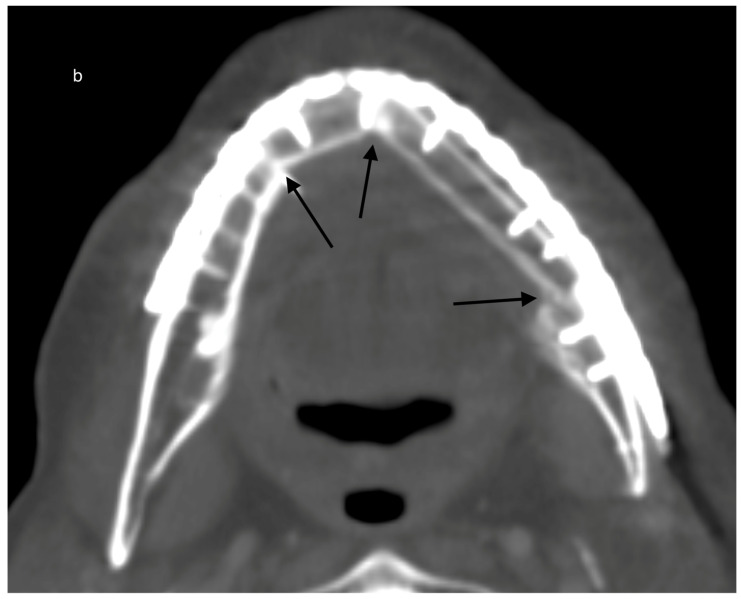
Example for ossification in CT scans over time in a case with a primary reconstructed mandible after malignant tumor resection and post-reconstructive adjuvant radiotherapy (postORT): Example for a reconstructed mandible with a 2-segmented fibula 265 days after operation. All three contact points are marked with black arrows (a). Slow progression of ossification (black arrows) after 494 days (b).
